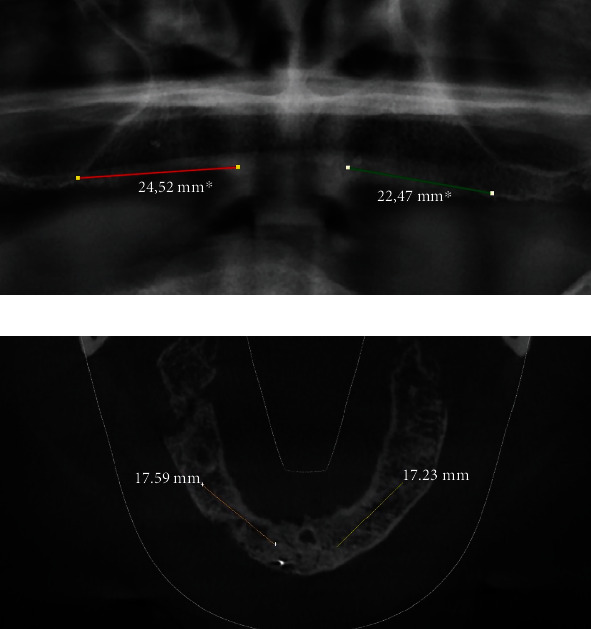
Measurement of the shortest vertical distance between the maxillary lateral incisor tooth region and the maxillary first molar tooth region on a panoramic image (a) and a cone beam computed tomography image (b).
